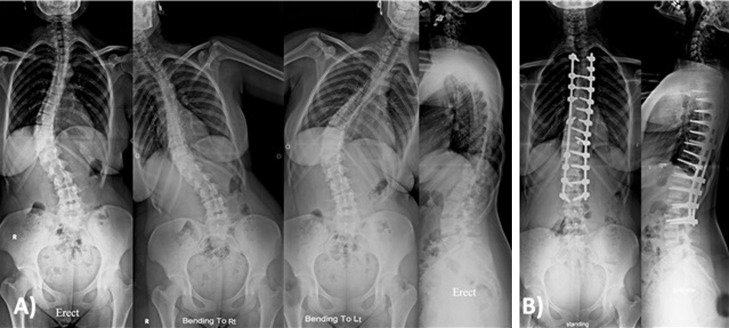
Female patient 18 years old with Lenke 1 c n. (A) Preoperative X-rays showing main thoracic curve of 55° that was corrected to 15° in side bending films with thoracic kyphosis of 38°. (B) Two years postoperative X-rays showing posterior correction and fusion from T4-L3 with main thoracic curve correction to 5° with thoracic kyphosis of 26°.
